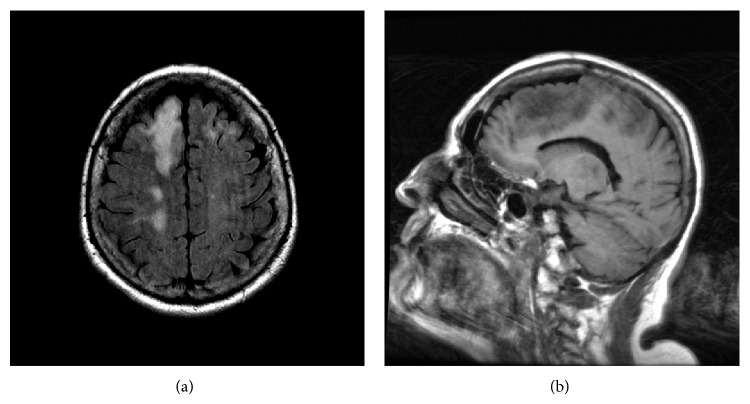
(a) T2 FLAIR: confluent hyperintense lesion in R hemisphere over R frontal region, R motor cortex. Involvement of U fibers. Minimal perilesional edema. Few lesions are seen in left frontal region as well. (b) Sagittal T1 images show hypointense lesion in the left frontal regions corresponding to the axial images.
